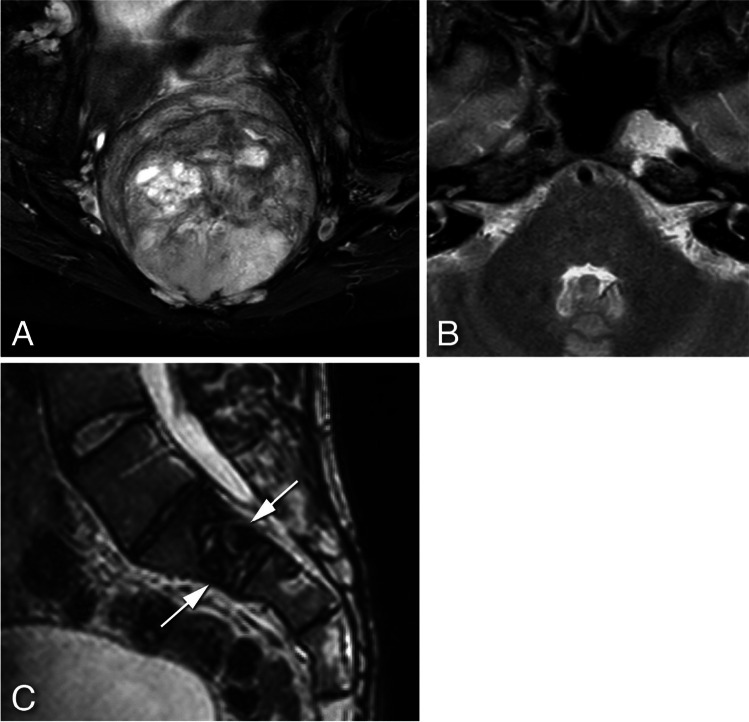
Examples of signal intensity. A- Heterogenous *hyperintensity* seen on T2-weighted MRI sequence in a midline located chordoma of the sacrum with extraspinal extension. B- Homogeneous *hyperintensity* seen on T2-weighted MRI sequence in chondrosarcoma of the skull base. C- *Hypointensity* seen on T2-weighted MRI sequence in a midline located sacral giant cell tumor of the bone with intraspinal extension
